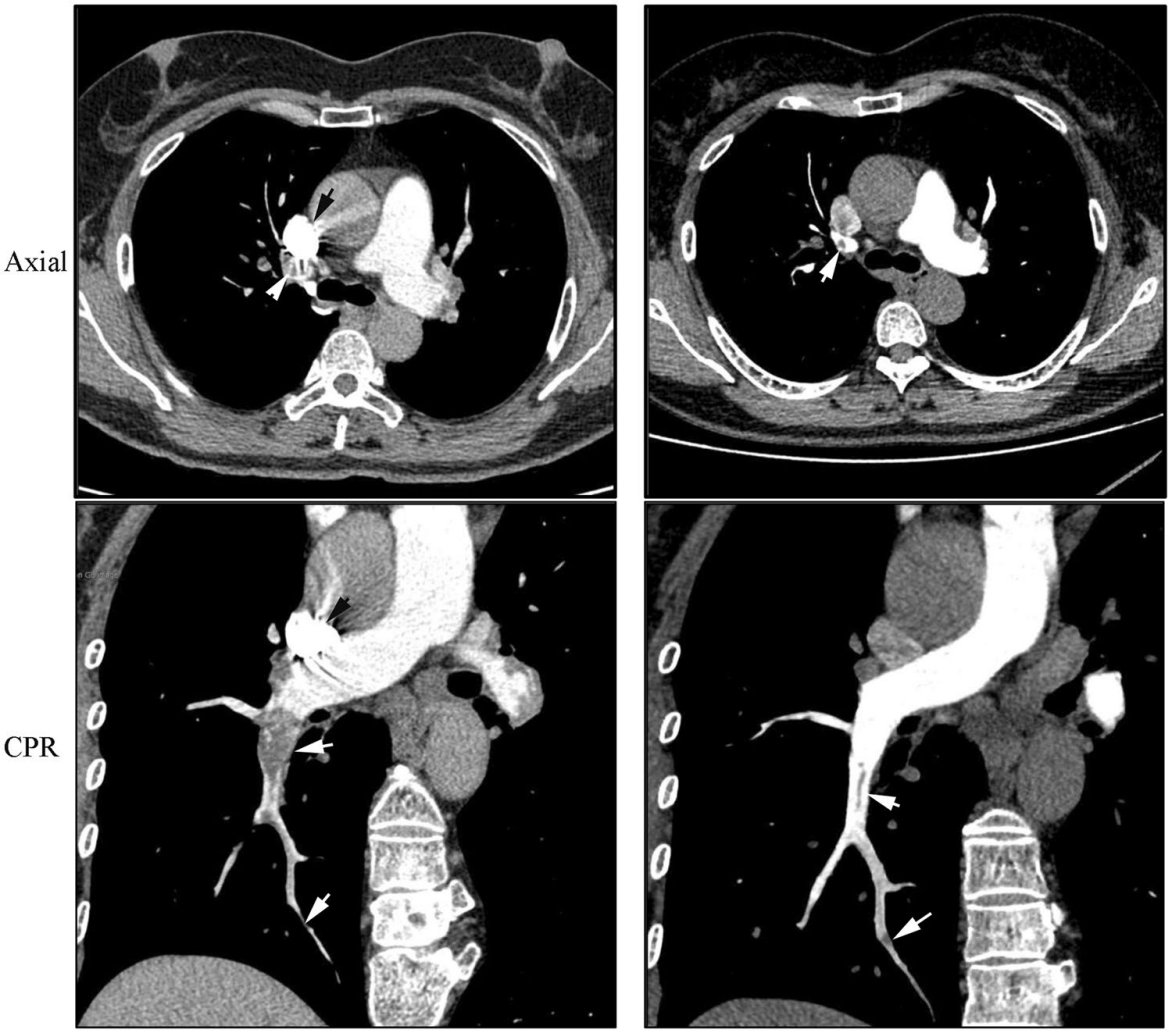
A 58-years old female patient who underwent computed tomography pulmonary angiography (CTPA) examinations both using test bolus method (Left) and the optimized protocol (right). She firstly underwent the CTPA using test bolus method. The artifacts of superior vena cava (SVC) were occurred (black arrow) in axial and curve plane reformation (CPR) images which influenced embolism detection (arrow head). During the follow-up at 6th month, the CTPA was performed using the optimized protocol (biphasic time-enhancement curve). No artifacts of SVC was observed. The two methods both detected the pulmonary embolism at lobar PA and segmental PA (white arrow).
